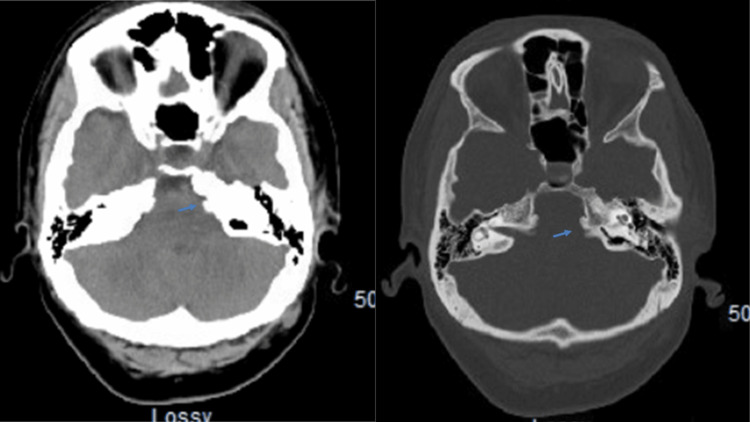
Preoperative axial head CT The view shows a thickened left petrous bone (blue arrow) CT: computed tomography
